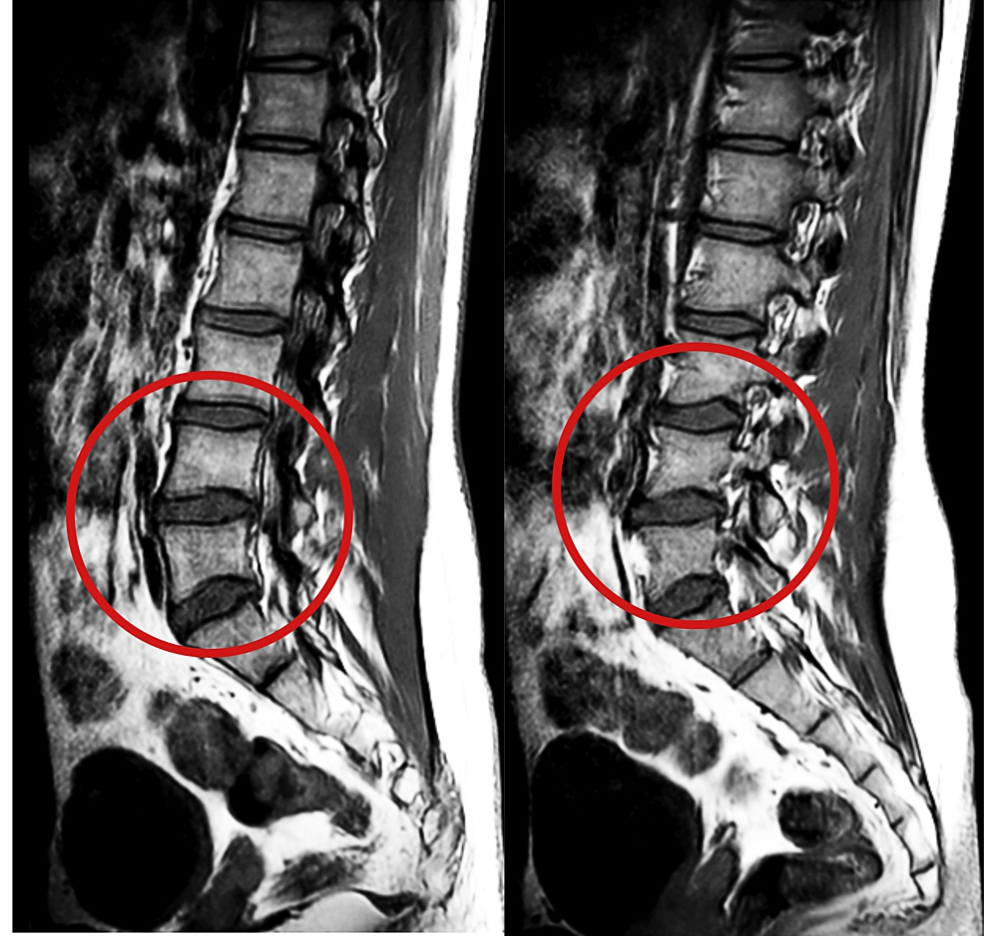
MRI of the lumbar spine. MRI: Magnetic resonance imaging. Bulging disc at the following levels: L3-L4 and L4-L5.
